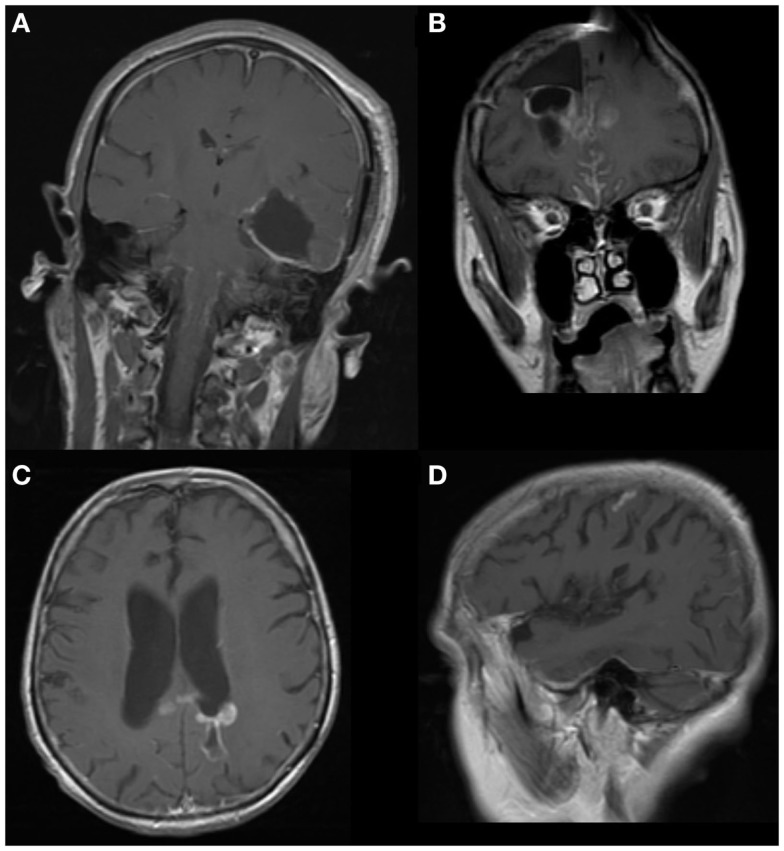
MRIs (1.5T, T1-weighted, post-contrast). (A) GBM spread along cerebral cortex and caudally to brainstem. (B) GBM with spread to interhemispheric fissures and a new parenchymal focus in the contralateral hemisphere. (C) GBM adjacent to left lateral ventricle. (D) Leptomeningeal spread from (C) to a cortical sulcus on the same side. This was not apparent on axial imaging.
